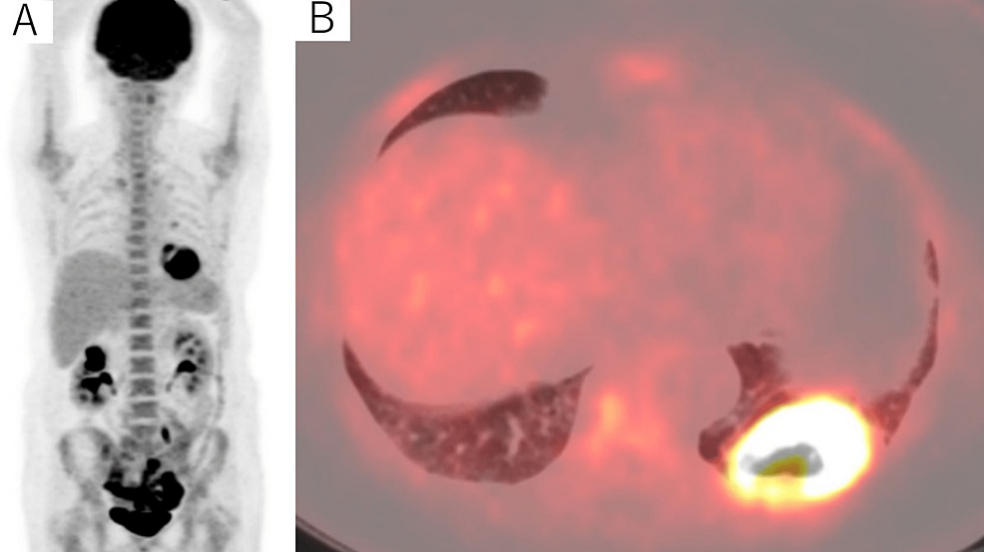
Representative images of FDG PET/CT (A) Maximal intensity projection image of whole-body 18F-FDG uptake; (B) Axial plane of the fusion PET/CT image 18F-FDG: fluorine-18-fluorodeoxyglucose; PET: positron emission tomography
